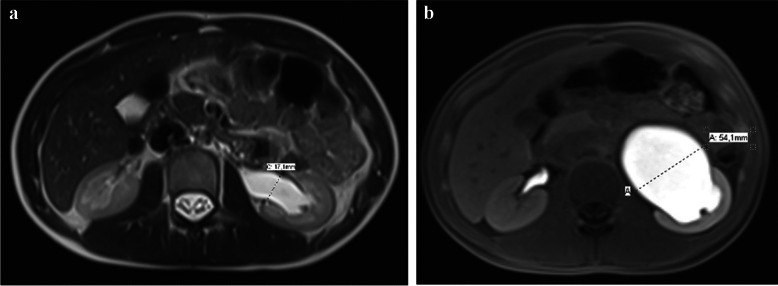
Measurements of the anteroposterior diameter of the renal pelvis before and after furosemide injection in a 14-year-old girl with left-sided ureteropelvic junction obstruction. a Axial T2-weighted MRI image before furosemide injection. b Axial fat-saturated T1-weighted MRI image after intravenous injection of furosemide and a gadolinium-based contrast agent* (images from the pediatric imaging department of Lille University Hospital)*
